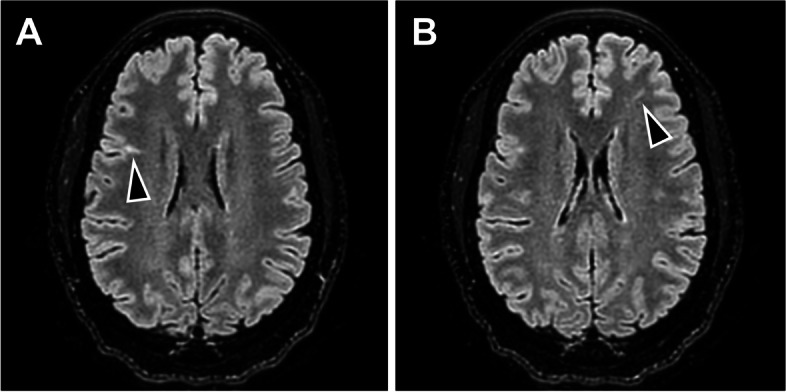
Tubers on MR images. (A & B) Two small, focal cortical tubers in the frontal lobes were identified on brain MRI. The patient did not exhibit clinical symptoms, including a history negative for seizures and learning delay. The tubers are delineated with the arrow
